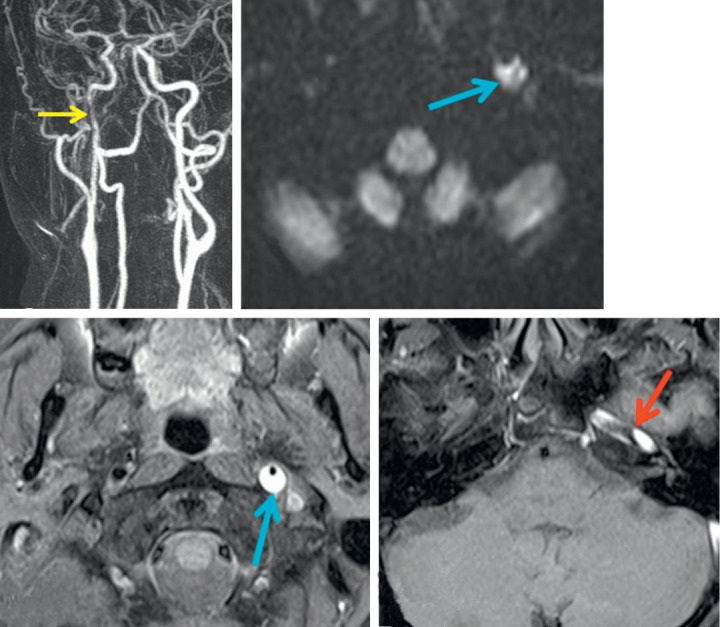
Angio MRI of the supra aortic trunks (a); MRI with diffusion slices B1000 (b); T1 gadolinium FATSAT (c,d); Carotid hematoma (c) with carotid stenosis (a). Carotid dissection with intracranial extension (d) (from Vignal-Clermont C, Tilikete C, Milea D. Neuro-ophtalmologie. 2e edition, 2016, Elsevier)
